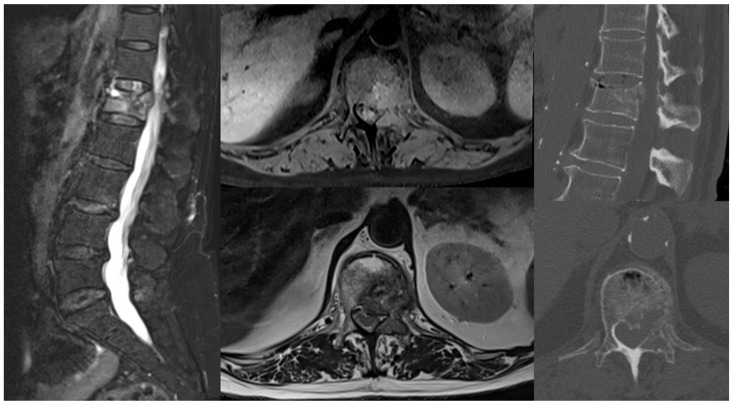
Pre-operative MRI and CT assessment. L1 pathologic fracture with epidural spinal cord compression of Bilsky grade 2.
